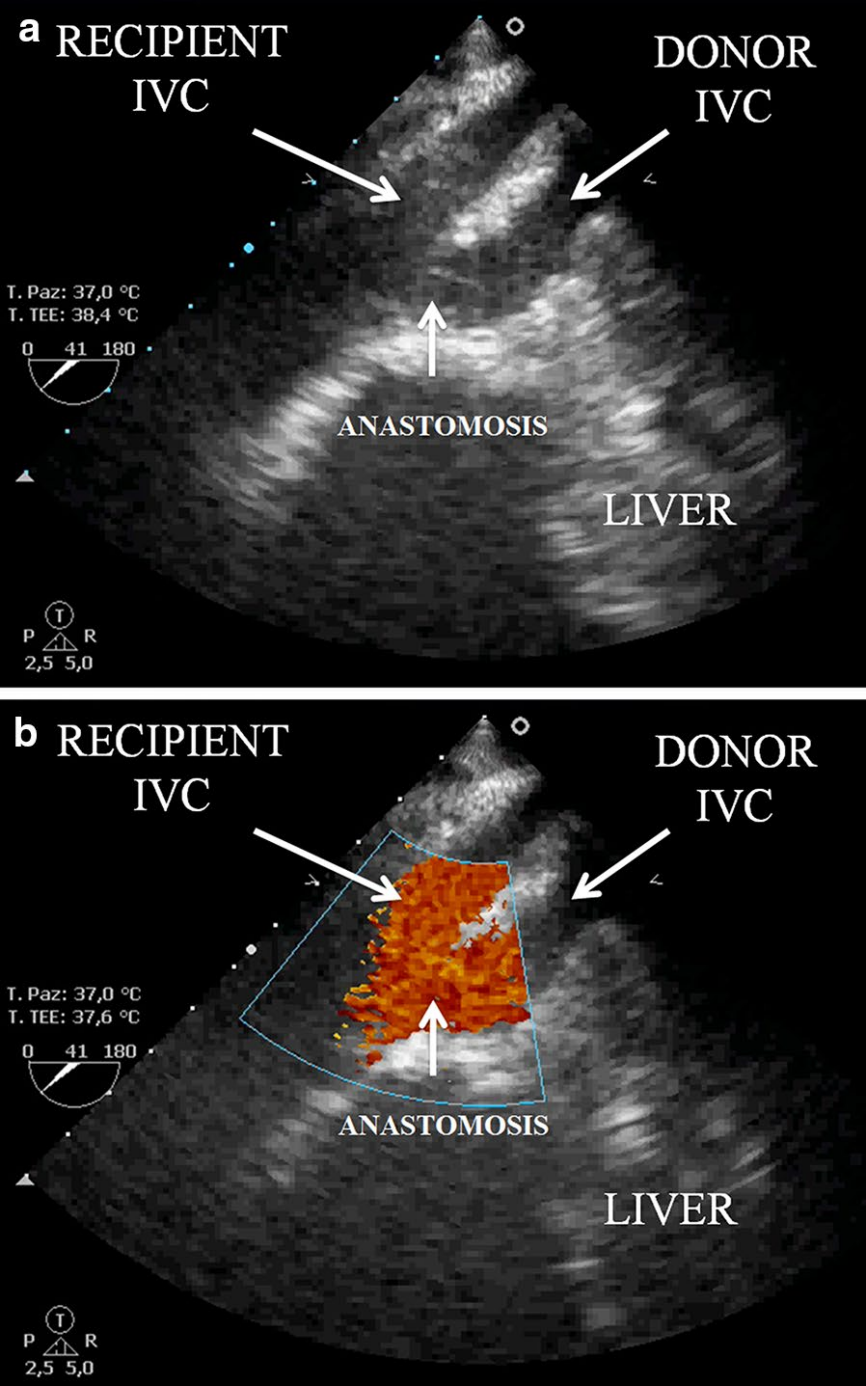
Transesophageal transgastric view at 41°, showing the double inferior vena cava in parallel manner without (a) and with (b) Doppler color flow through the patent terminolateral cavo-cavostomy
